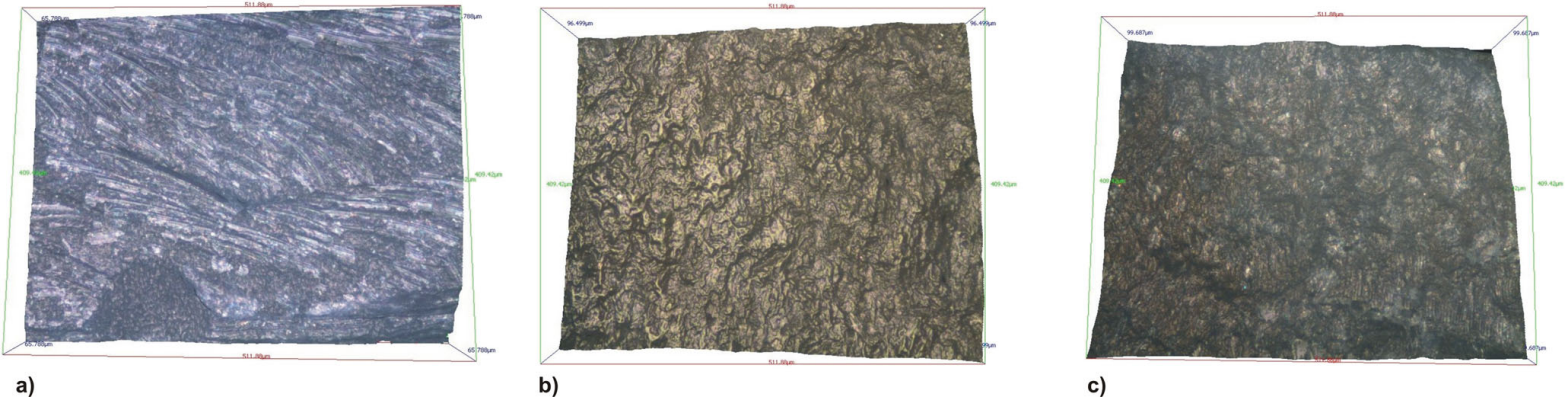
The different patterns of proximal box floor surface roughness after the use of the proximal box finishing methods with three different instruments: a rotating, b sonic, and c ultrasonic
